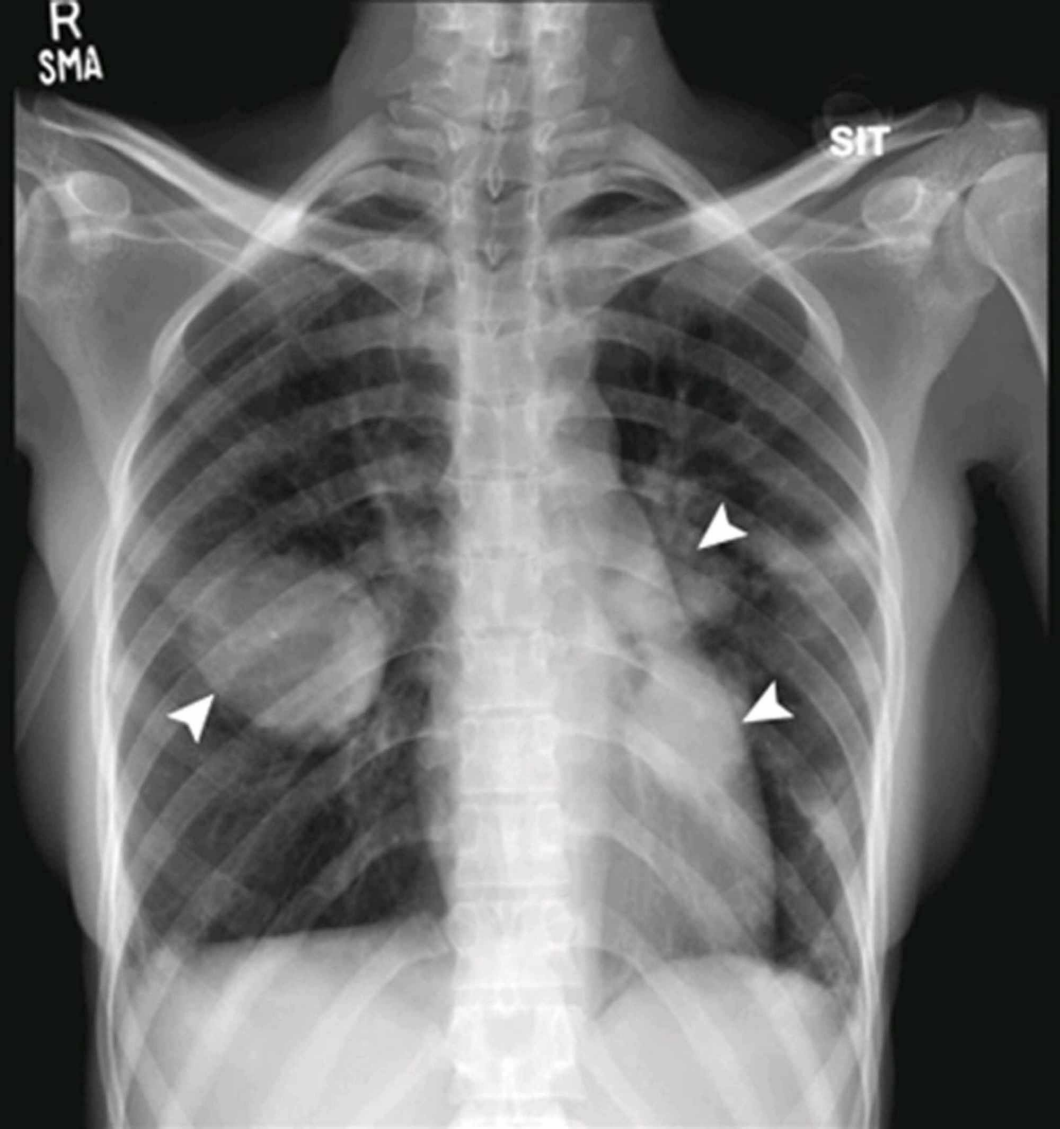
Bilateral perihilar rounded opacities were noted on initial chest x-ray (arrowheads).
